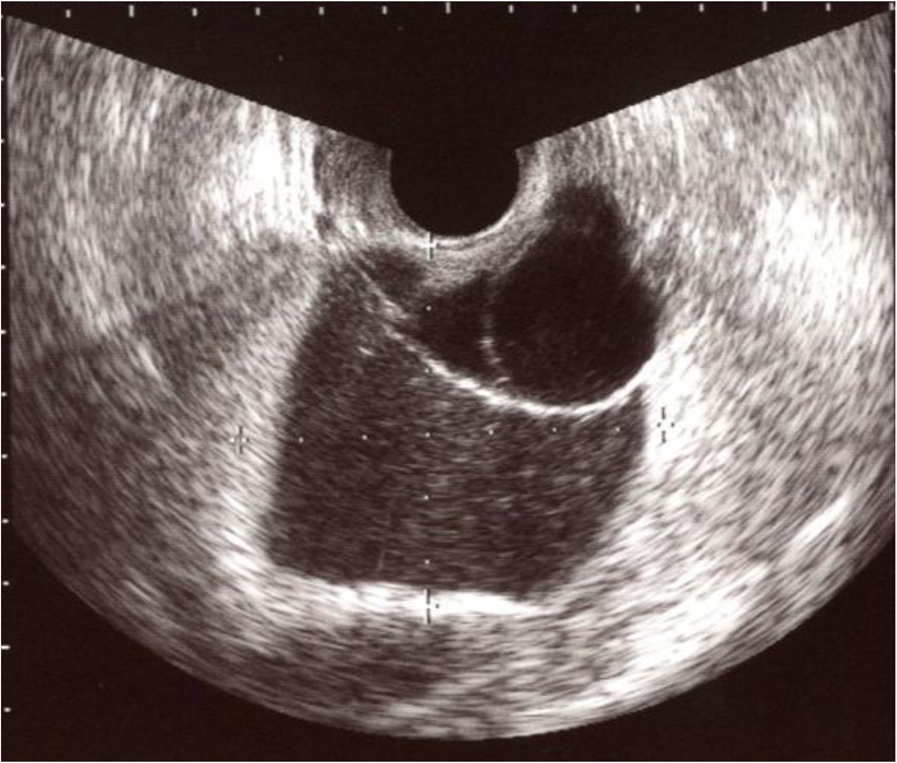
Transvaginal ultrasonography. Transvaginal ultrasonography showed a multilocular cystic lesion without a solid part in the pelvic cavity.
